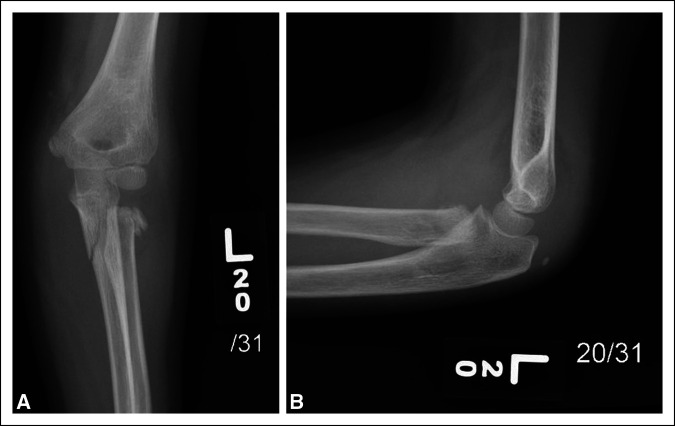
Radiographs of AP and lateral views of the left elbow showing proximal ulna and proximal radius with radial neck fracture.
